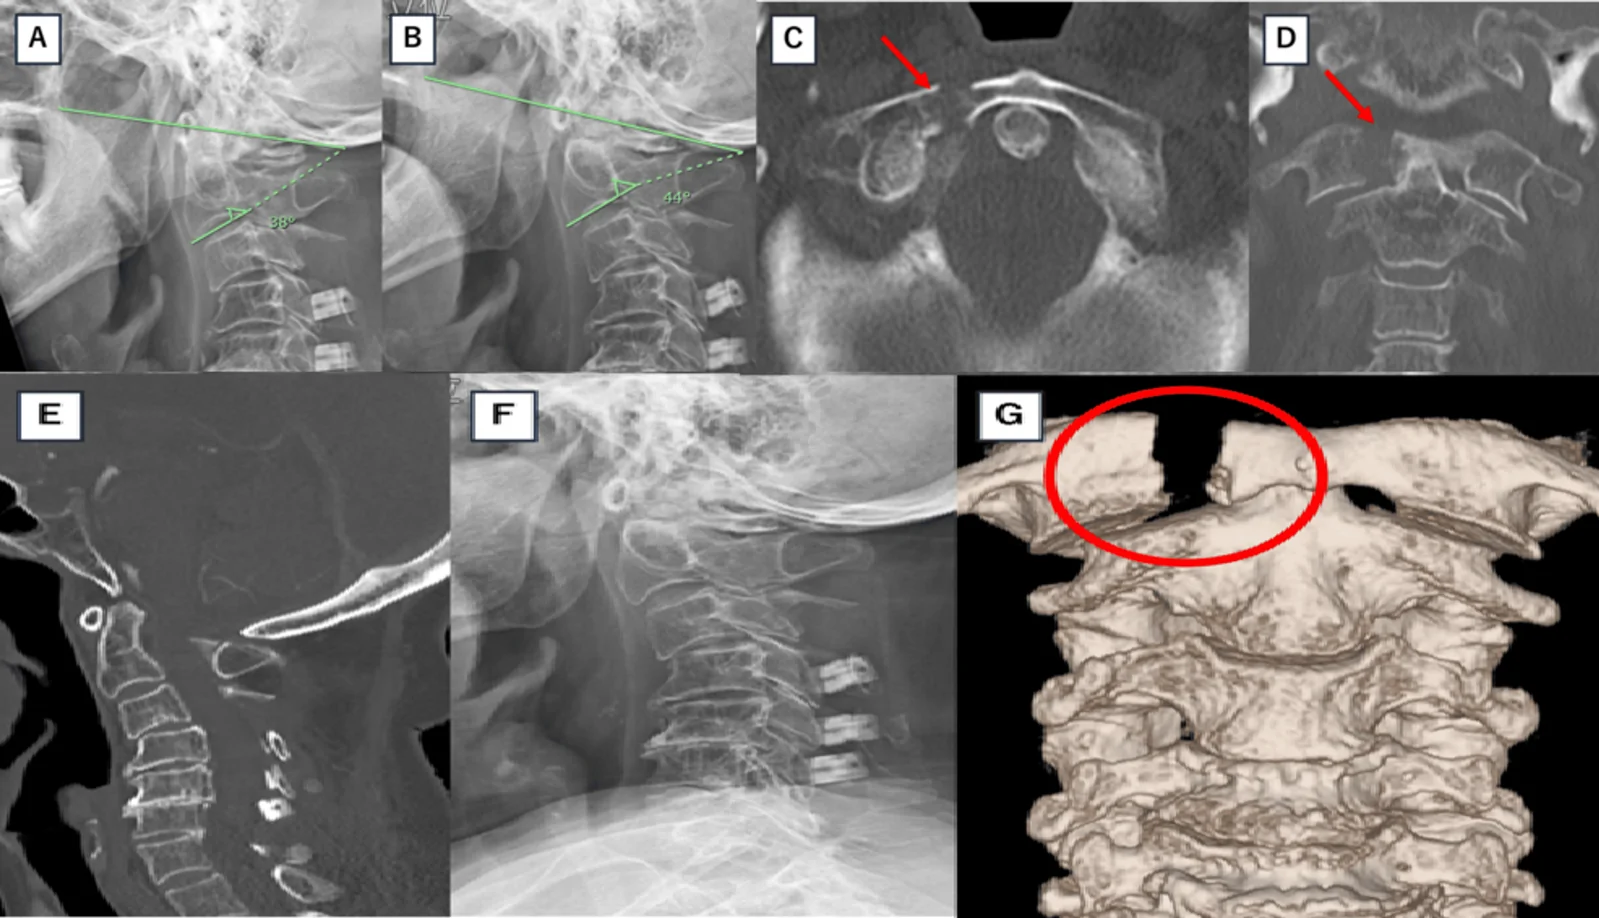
Progression of fracture displacement and lateral mass subluxation (A) Prefracture occiput-C2 angle measured at 38°. (B) Postfracture occiput-C2 angle increased to 44°. (C) Axial CT image demonstrating progression of anterior arch fracture. (D) Coronal CT image demonstrating lateral mass displacement. (E) Sagittal radiograph obtained during follow-up. (F) Lateral radiograph showing change in occiput-C2 alignment. (G) Three-dimensional reconstructed CT image CT: computed tomography
